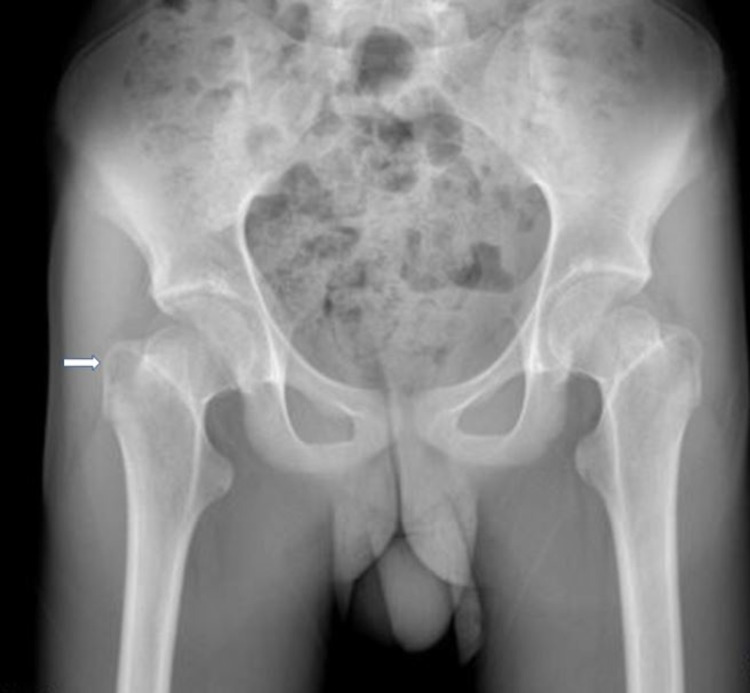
X-ray of the pelvis The image shows a subtle, non-specific, and well-defined lucency in the right trochanteric region (white arrow)
